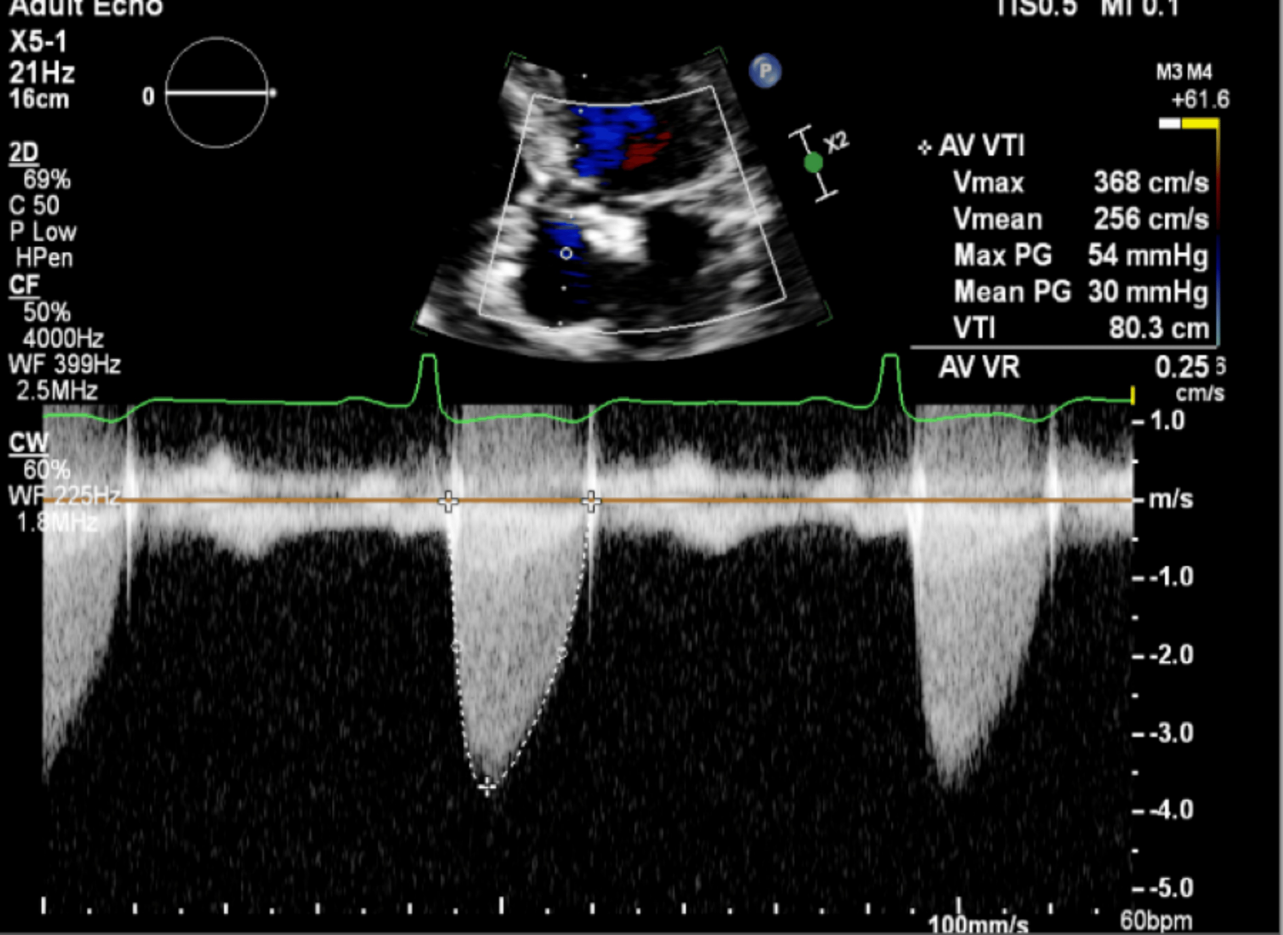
Continuous-wave Doppler interrogation across the aortic valve demonstrates an early-peaking systolic flow pattern with a mean transvalvular gradient of 30 mmHg, consistent with moderate aortic stenosis. The contour of the Doppler envelope raises concern for additional subvalvular obstruction, supporting suspicion of a subaortic membrane contributing to LVOT obstruction X2 denotes a two-fold zoom applied by the echocardiography machine, indicating image magnification rather than an anatomical distance measurement. LVOT: left ventricular outflow tract
